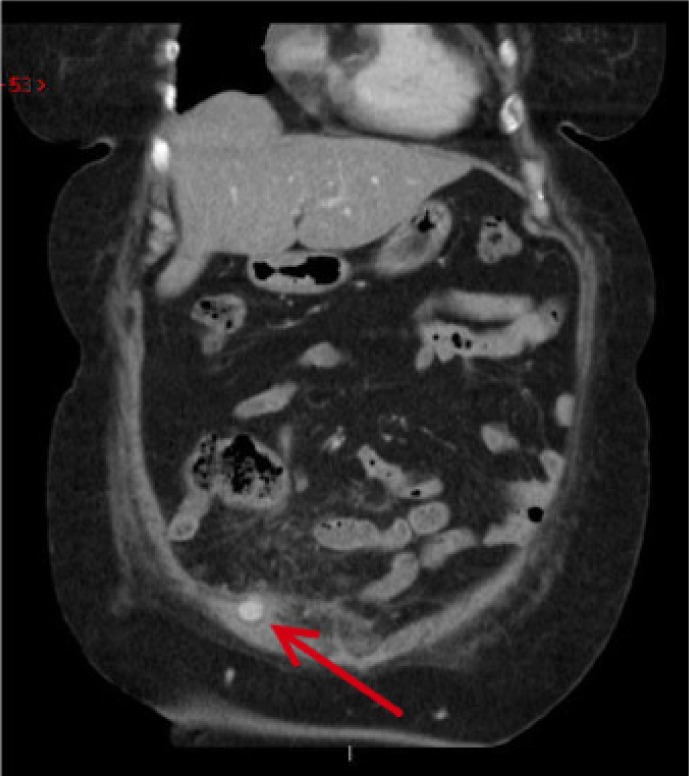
Intravenous contrast computed tomography revealing right inferior epigastric artery pseudoaneurysm (arrow)
